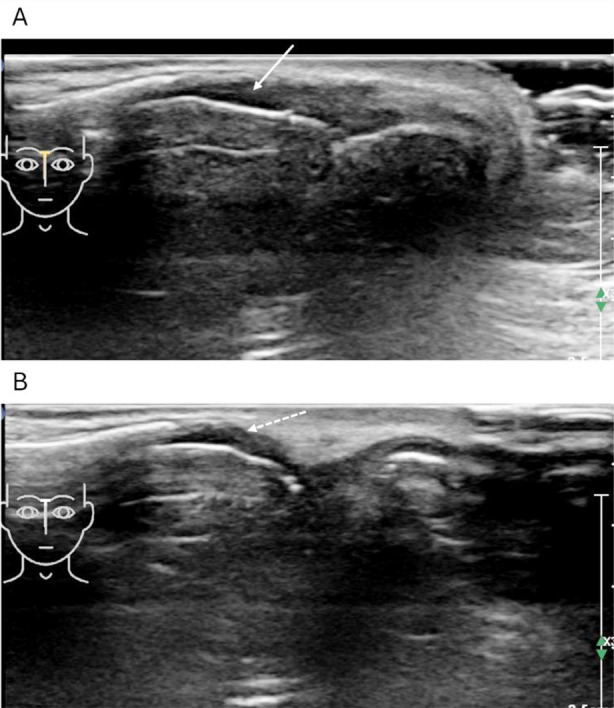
Ultrasound image of a 44-year-old male patient with incomplete fracture. A: The hyperechoic zone in the bone cortex of the nasal bone is continuous, slightly angled without displacement. Irregular and uneven echo areas can be seen in the superficial subcutaneous soft tissue (solid arrow). B: Two weeks later, the reexamination showed that the hyperechoic zone in the bone cortex of the nasal bone was continuous, slightly angled without displacement and the uneven echo area of the superficial subcutaneous soft tissue disappeared (dotted arrow).
